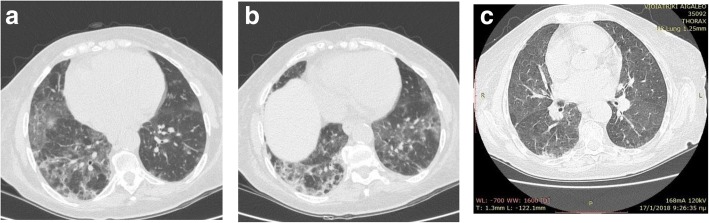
Computed tomography images of a 76-year-old female with lipoid pneumonia revealed ground-glass opacities and bronchiectasis in both lungs (a), (b). The shadows improved 2 years later (c)
